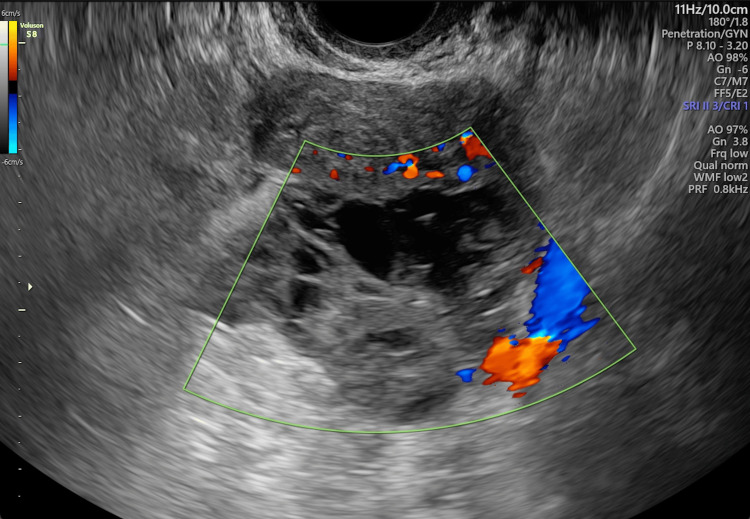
Ultrasound showing increased vascularity as well as organized tissue measuring 5 x 3.2 x 2 cm approximately
